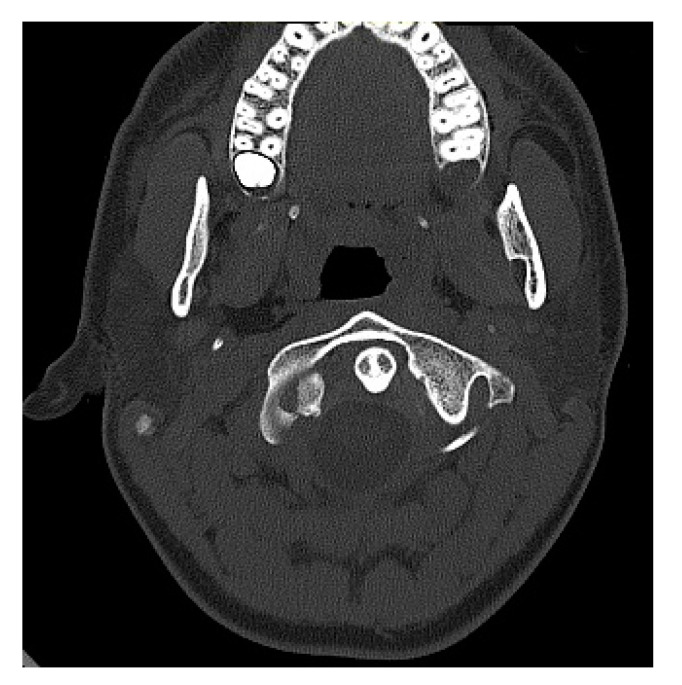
CT scan (transverse plane) of a boy aged 15.2 years (patient: P.P.) obtained after admission to the A&E room. Avulsed fragment of right condyle displaced medially by 4.0 mm. Dislocation of odontoid to the left.
